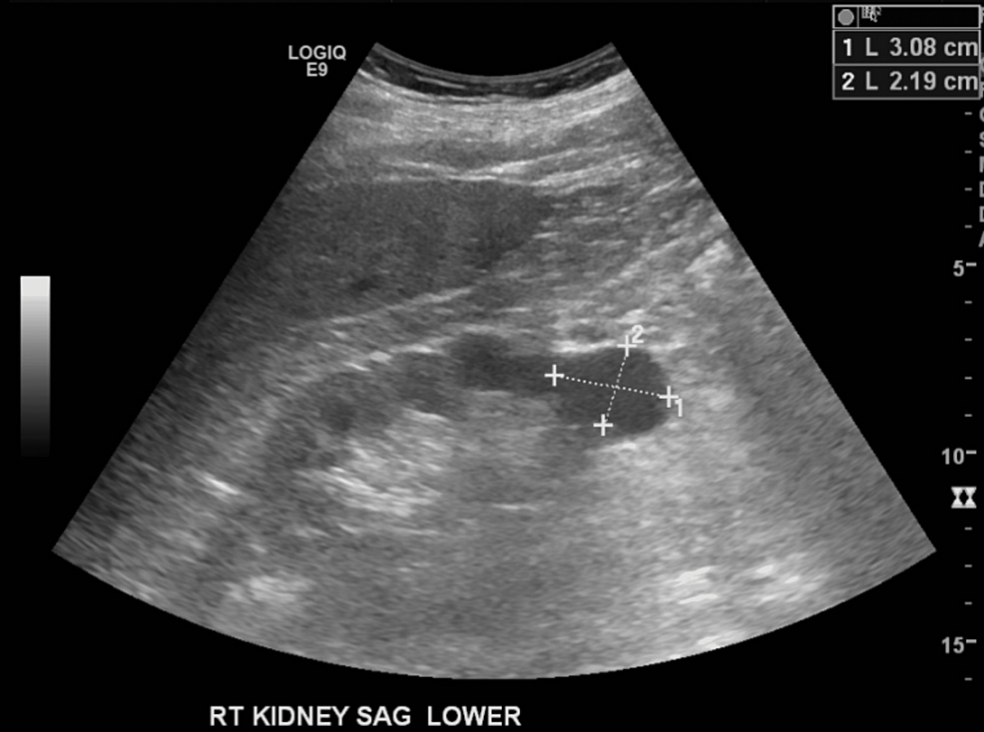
Renal ultrasound shows a simple cyst in the lower pole measuring 3.1 x 2.2 x2.3cm shown by 1 and 2. No evidence of mass, calcification, or hydronephrosis was noted.
